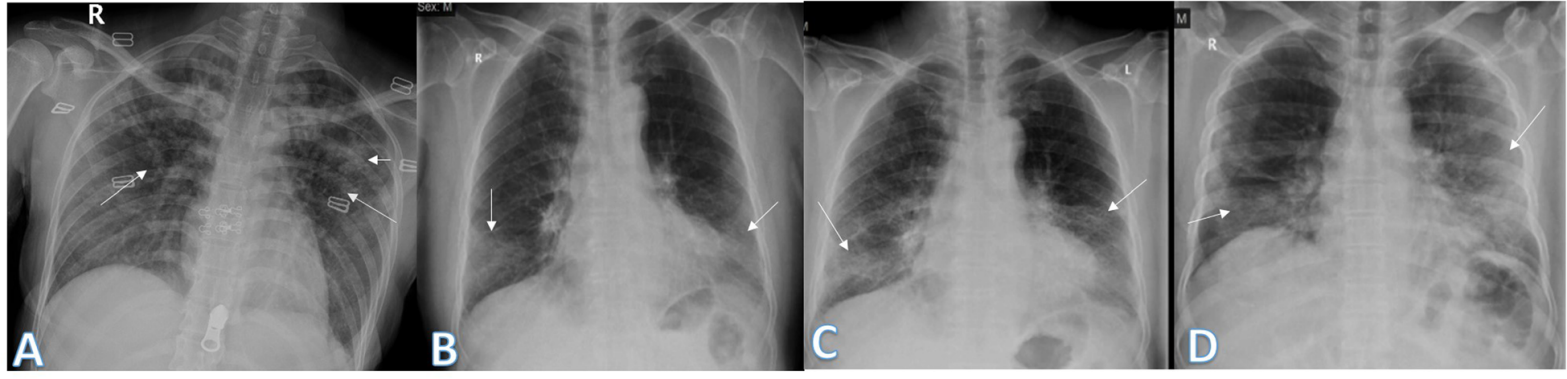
Four different patients with positive COVID-19. a 29-year female was complaining of cough and fever. Initial chest X-ray showed bilateral upper zonal ground glass opacities (long arrows) with small left upper zonal air space consolidation opacities (short arrow). The severity score was 2 for each lung so, TSS was 4. b, c 74-year male was complaining of dyspnea and fever. Initial and 1st follow-up chest X-ray showed bilateral lower zonal ground glass opacities (arrows). The total severity score was 3 in b and 4 in c. d 54-year male patient was complaining of SOB and cough. Initial chest X-ray show showed right lower zonal and left mid and lower zonal ground glass opacities (arrows). The severity score for the right lung was 1 and for the left lung was 3, so the total severity score was 4
